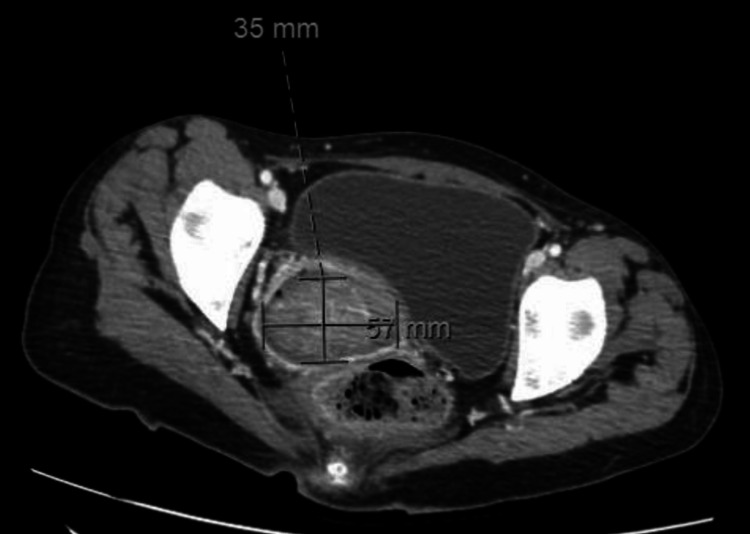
Computed tomography abdomen and pelvis (CTAP) showing complex mass, 5.7 x 3.5 cm hyperdense structure within the vaginal canal originating from the cervix, extending into the upper vaginal canal.
